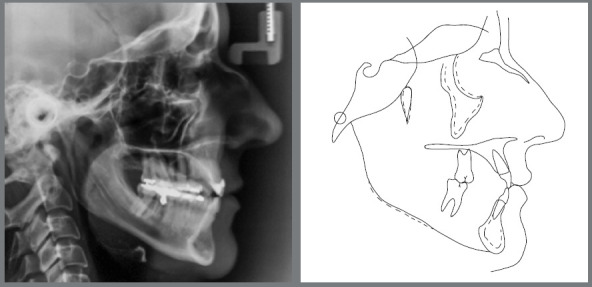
Baseline cephalometric radiograph and cephalometric
